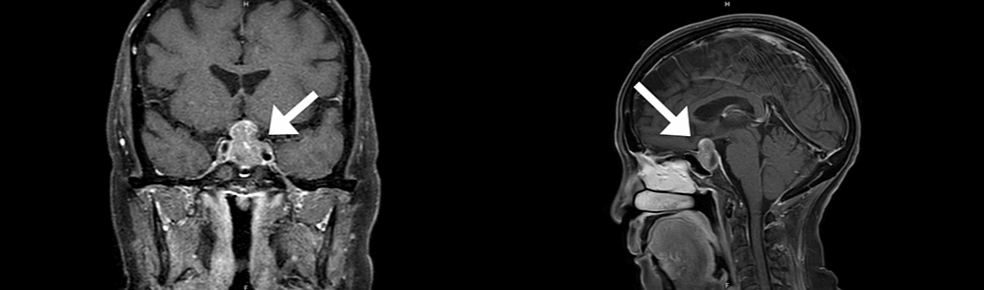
Coronal T1 (left figure) and sagittal T1 view (right figure) of magnetic resonance imaging confirming macroadenoma, compressing the optic chiasm and extending to suprasellar cisterns and the left cavernous sinus (arrow).
